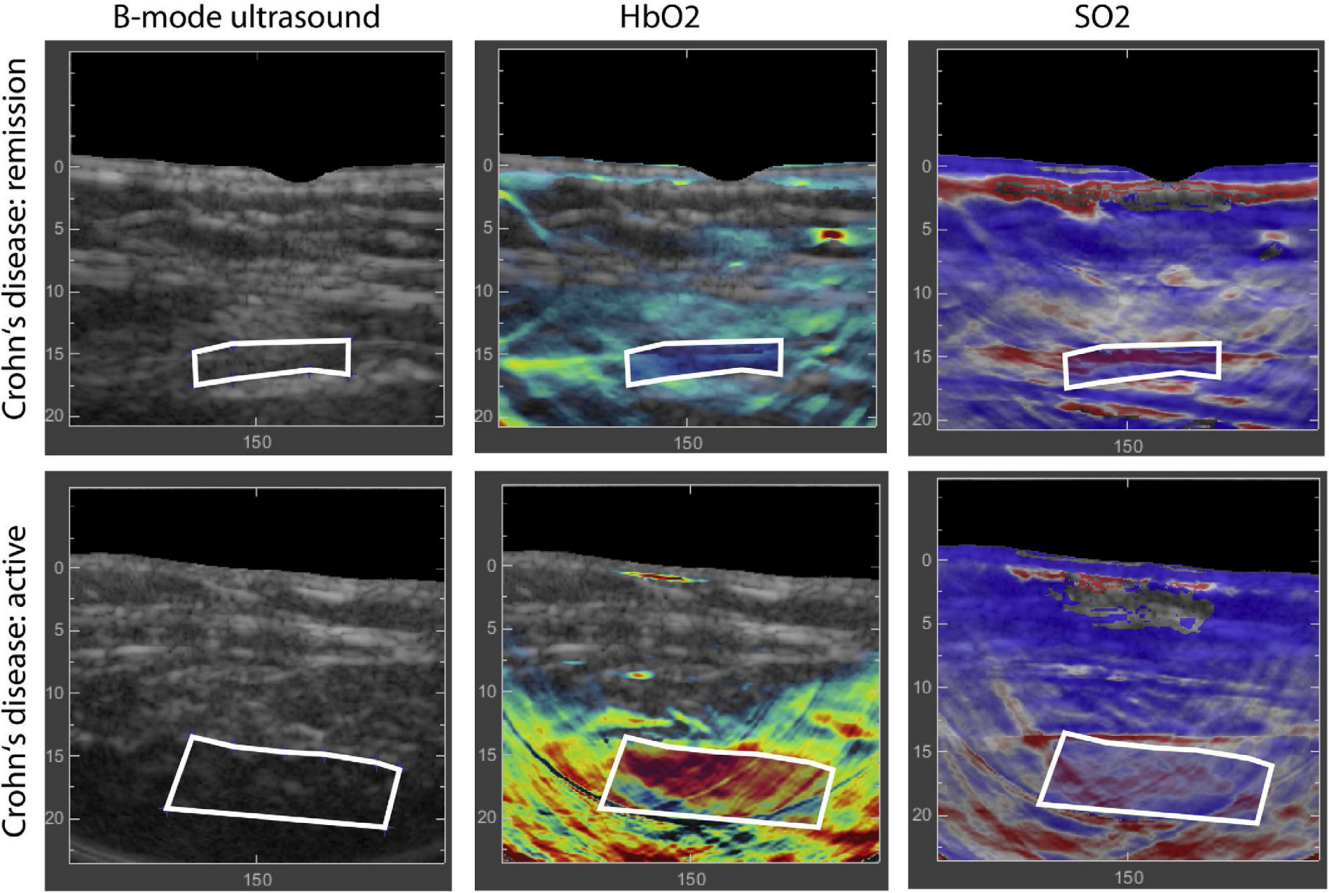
Representative MSOT images of the intestinal wall of a patient with Cohn’s disease (CD) in remission (upper panel) and a patient with active CD (lower panel). Identification of the intestinal wall was done using B-mode ultrasound image. It was observed that in contrast with the patient in remission, clinical and endoscopic CD activity was associated with increased signals for HbO_2_ and sO_2_ in the intestinal wall. Reprinted with permission from Ref. [122].
